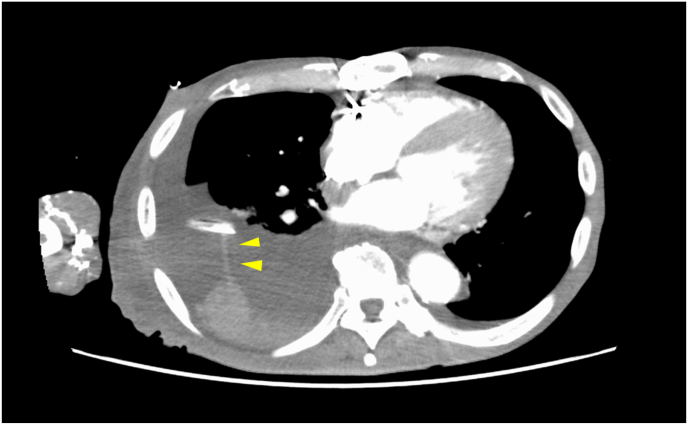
A contrast-enhanced CT scan showed that bleeding from the parietal pleura traveled along the first ICD and dropped into the intrapleural space.
